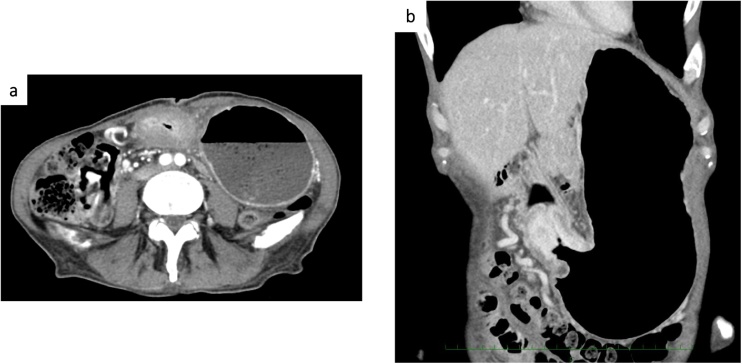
Enhanced abdominal computed tomography findings. Computed tomography revealed enhanced thickening of the stomach wall and pyloric stenosis.
